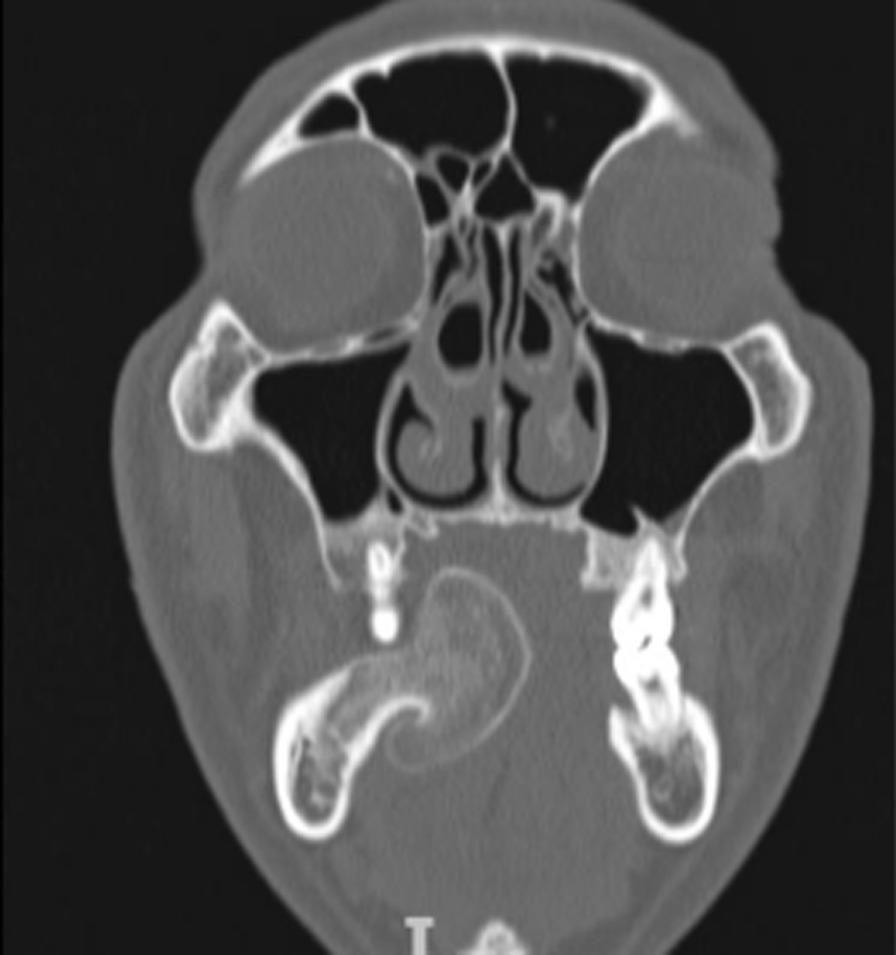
Coronal cut of the lesion in Computed Tomography image
